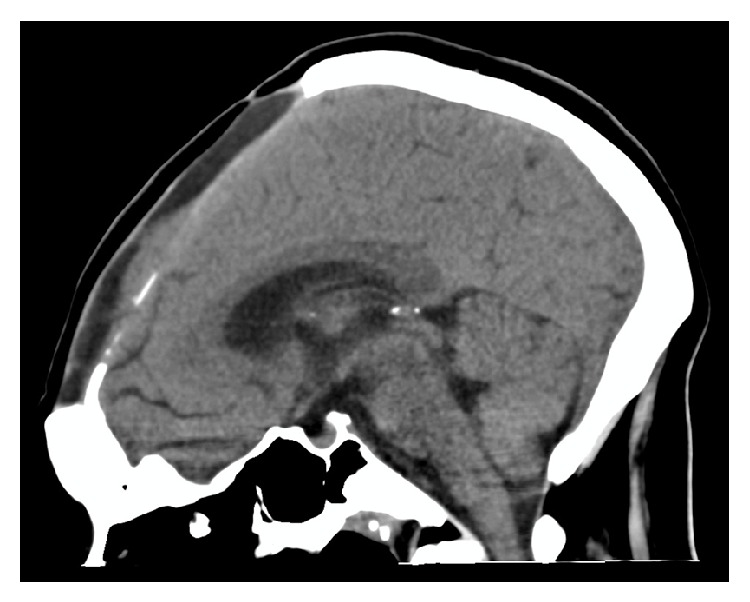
Sagittal CT demonstrating normal cerebellar tonsillar position after decompressive craniectomy.
